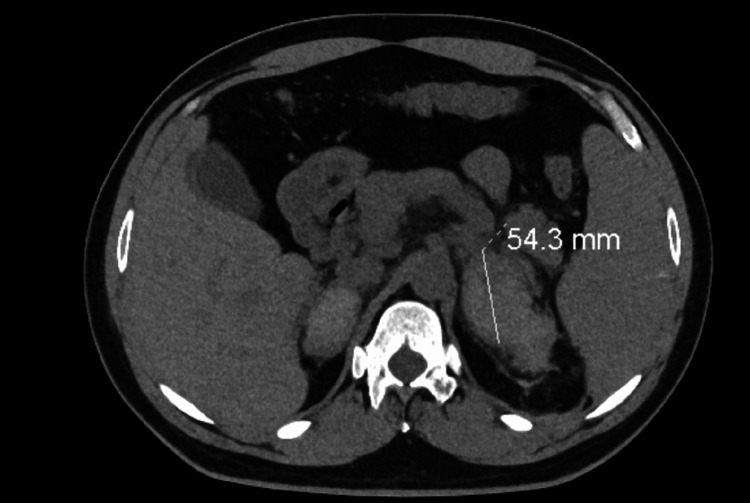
A transverse view of a non-contrast CT scan of the abdomen and pelvis shows a large left-sided adrenal hemorrhage.
